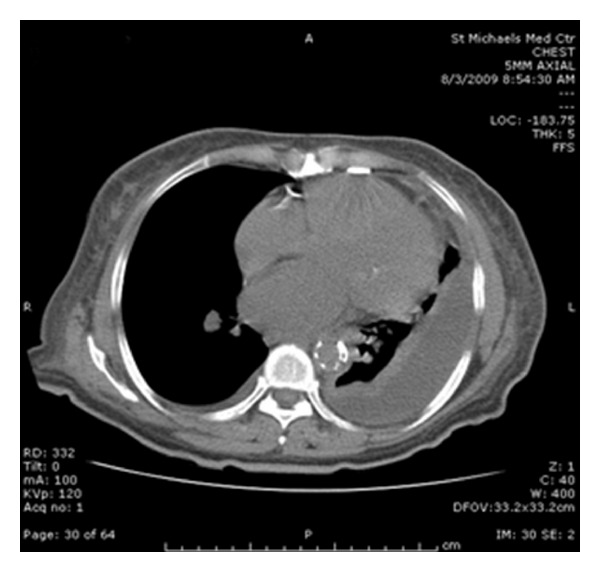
CT scan of the chest showing a large left pleural effusion with loculation.
